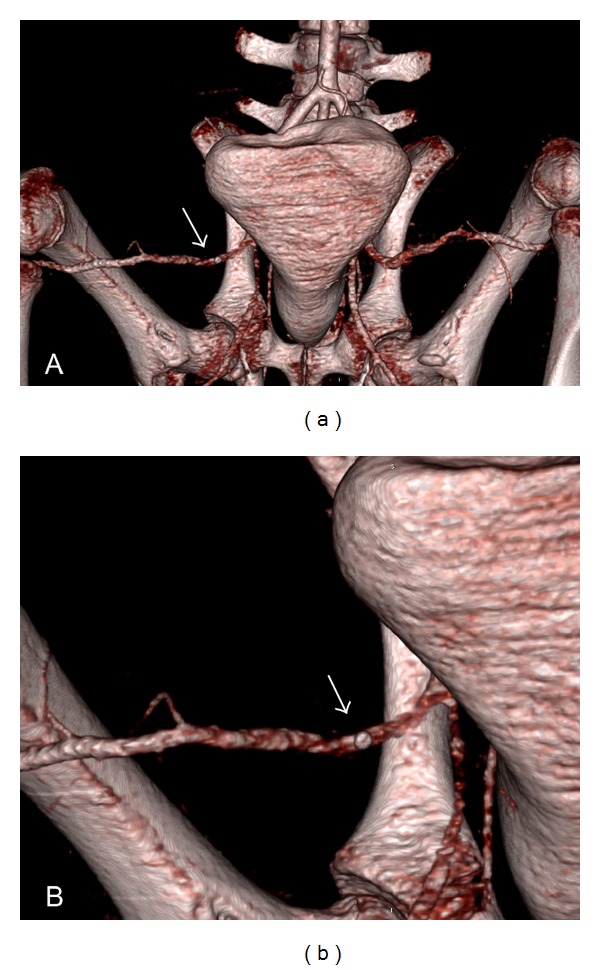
Three-dimensional visualization of a CT dataset in arterial contrast phase using volume rendering technique (VRT). (a) VRT after application of the VCD and (b) close-up of right FA with VCD clip in situ. Note unimpeded patency of the right femoral artery after VCD application. Note markedly different anatomy when compared to human vasculature.
